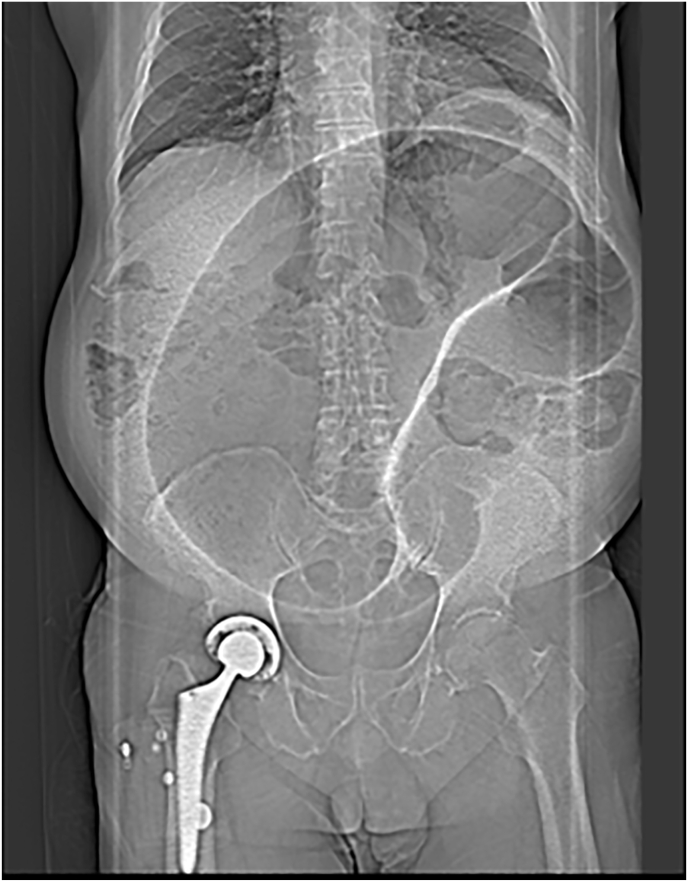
Sigmoid Volvulus anterior/posterior CT abdomen/pelvis.
